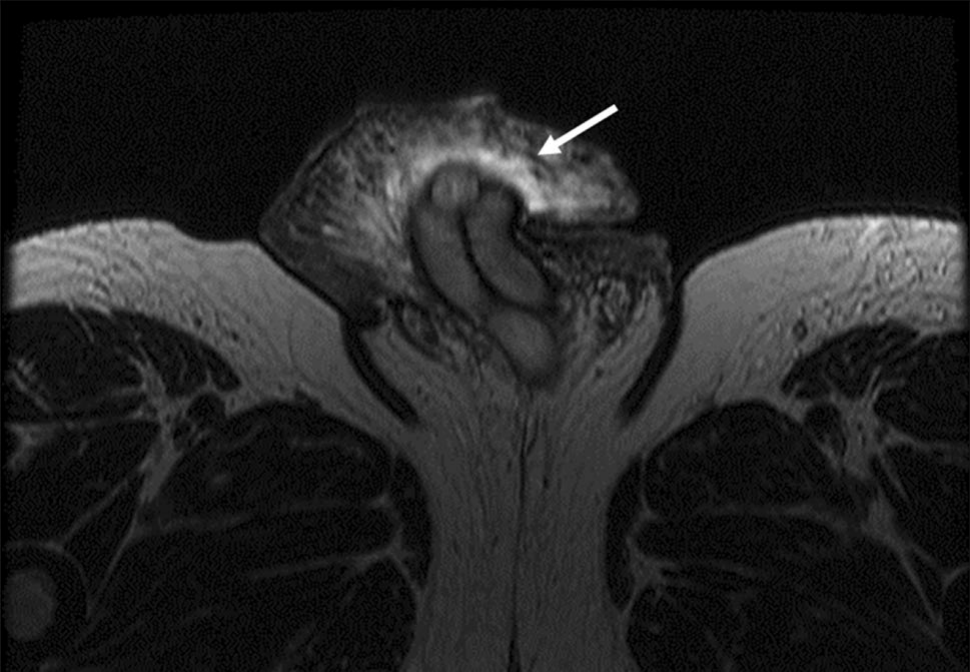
52-year-old man with KS and HIV. MRI of pelvis. T2-weighted axial image showing hyperintense thickened soft tissue in glans penis (arrow)
